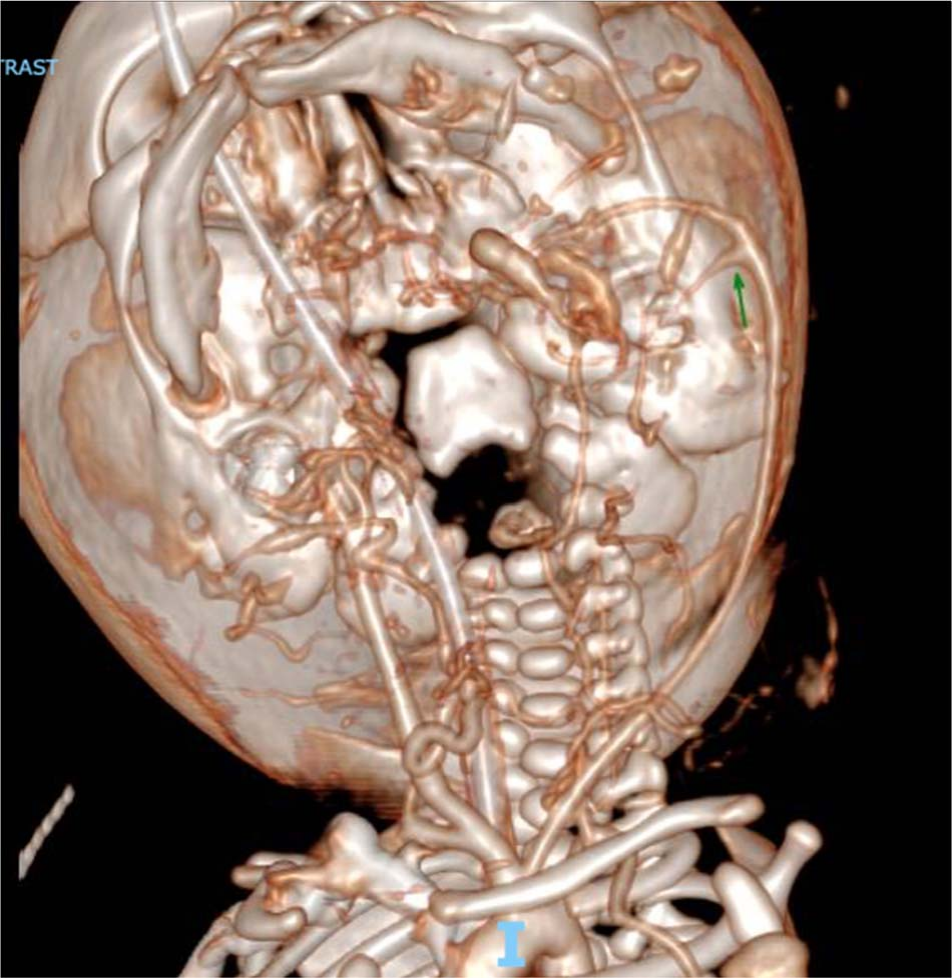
CT angiogram of the head with intravenous contrast demonstrating a hypervascular mass with arterial feeders originating from the left thyrocervical trunk, left external carotid artery, and right external carotid artery.
